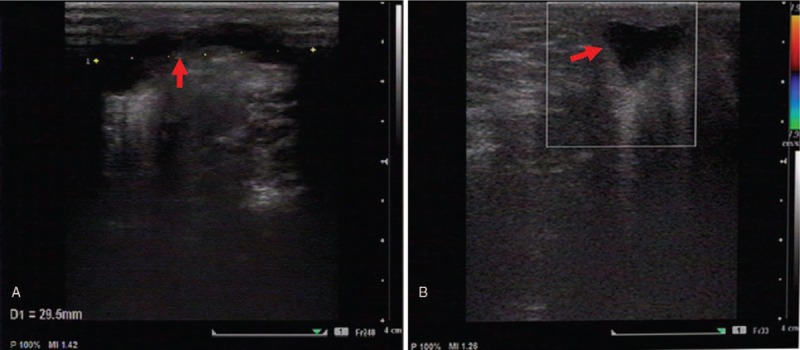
Magnetic resonance imaging (MRI) contrast before and after treatment: Before treatment, (A) axial T1-weighted, (B) axial T2-weighted, and (C) sagittal T2-weighted MR images show extensive exudation and irregular strip-like mixed signals (arrow) in the subcutaneous tissue of right buttock and the right thigh. After the treatment, (D) axial T1-weighted, (E) axial T2-weighted, and (F) sagittal T2-weighted MR images show that there was less subcutaneous soft tissue exudation in the right buttock and right thigh than before, and no obvious abnormal signal (arrow) was observed.
